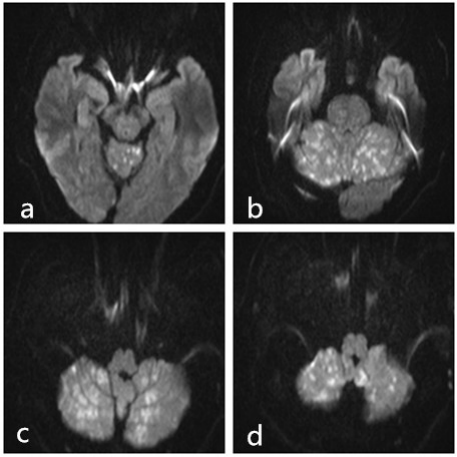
MRI showed multiple acute infarcts in the cerebellum.
